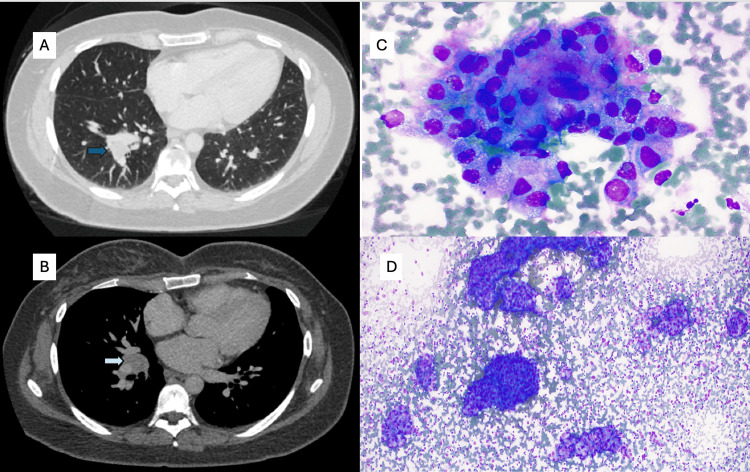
Thoracic and cytologic findings of metastatic meningioma. (A) Axial chest CT demonstrating a right lower-lobe pulmonary nodule (blue arrow) corresponding to a biopsy-proven metastatic meningioma.
